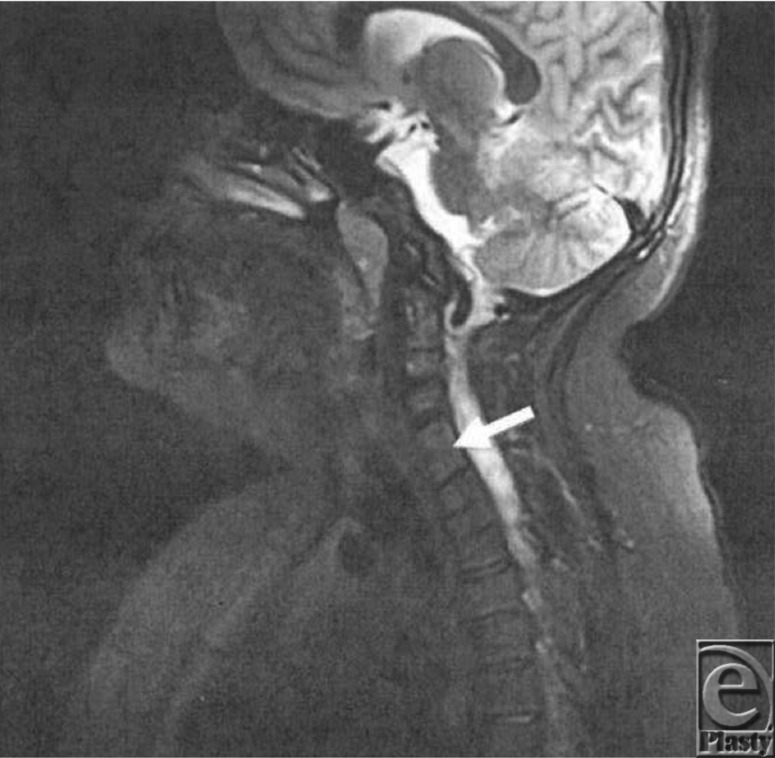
Sagittal plane, T2-weighted sequence with contrast showing segmentation anomalies of cervical vertebrae.
